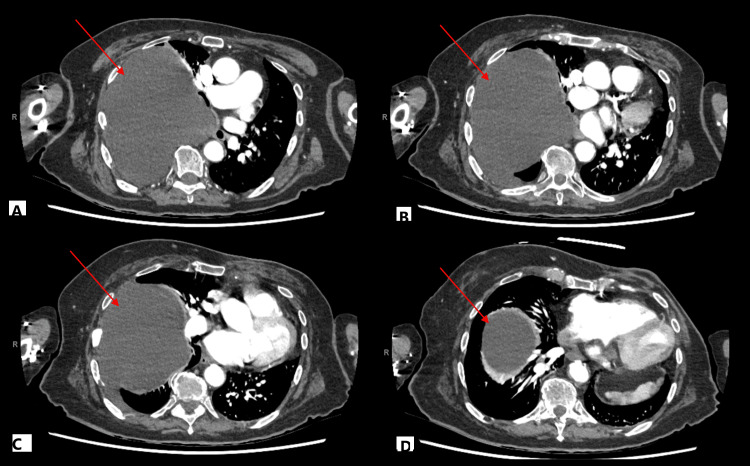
Selected axial CT images of the thorax
